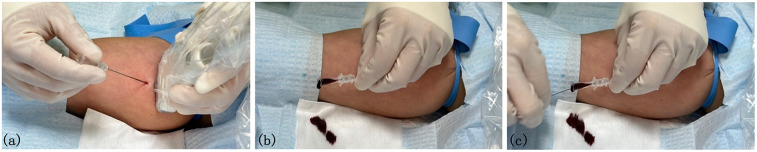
Convectional puncture group (the operator performs puncture in the lateral position without using the guide needle holder, a→b→c). (a): Hold the needle in the right hand for puncture, hold the ultrasound probe in the left hand, and puncture at an angle of approximately 45°. (b): If the puncture is successful and blood returns is seen, change the left hand to fix the puncture needle and take the guide wire with the right hand. (c): The right hand slowly pushes the guide wire through the end of the needle.
